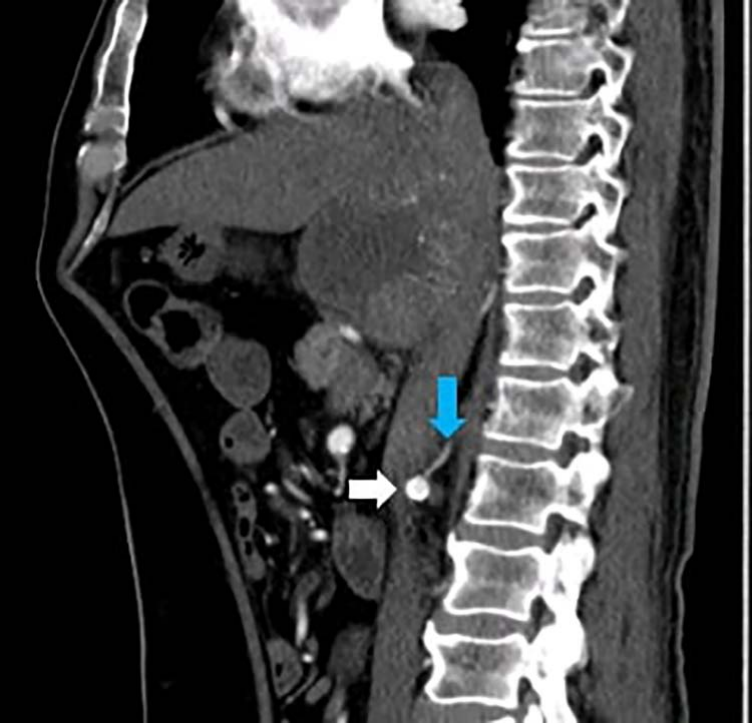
CT scan sagittal section arterial phase showing a branch (blue arrow) originating from the right renal artery (white arrow) supplying the lesion in Segment I of the liver.
